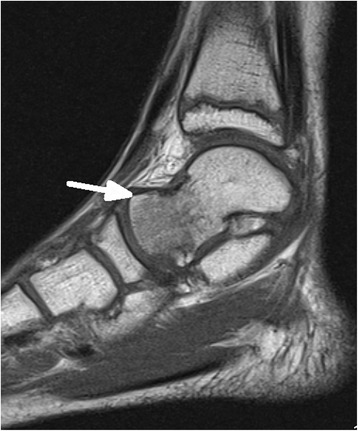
Sagittal T1-weighted sequence did´nt showed a fracture
